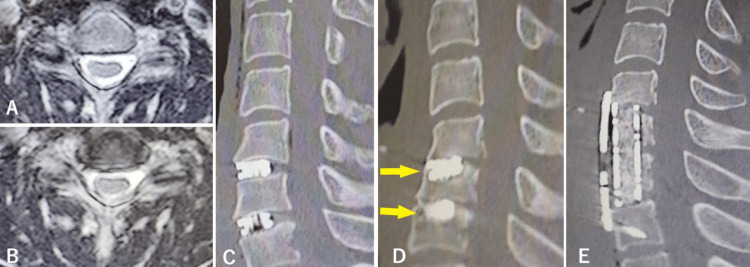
Case 2: Sagittal MRI and CT scan before and after two operations. T2-weighted MRI axial image before the first operation at C5/6 (A) and C6/7 (B), sagittal reconstructed CT scans after the first operation showing that sufficient decompression was achieved (C), 37 days after the operation showing that the cage at C5/6 had subsided by 7 mm and the cage at C6/7 had subsided by 6 mm (D, arrows), and after the second operation showing the proper position of the mesh and plate and good cervical spine alignment (E). MRI: magnetic resonance imaging
